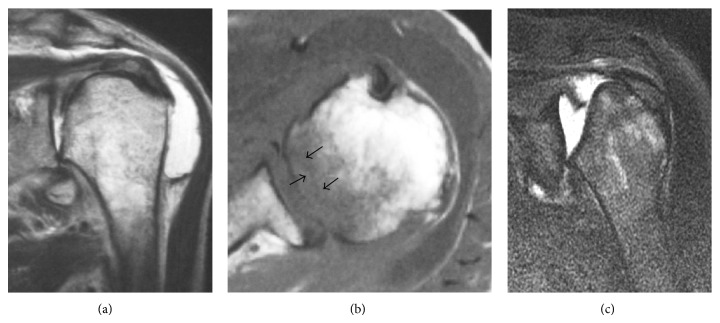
Case  2. Magnetic resonance image (MRI) of the left shoulder at symptom onset (a, b) and at the first visit to our hospital (c). (a) T2-weighted MRI (oblique coronal plane) demonstrates joint effusion and a slightly collapsed humeral head. (b) Axial, T1-weighted MRI shows a subchondral serpiginous pattern of low signal intensity with associated bone marrow edema. (c) T2-weighted MRI (coronal view) demonstrates the collapse of the humeral head and an intact rotator cuff.
